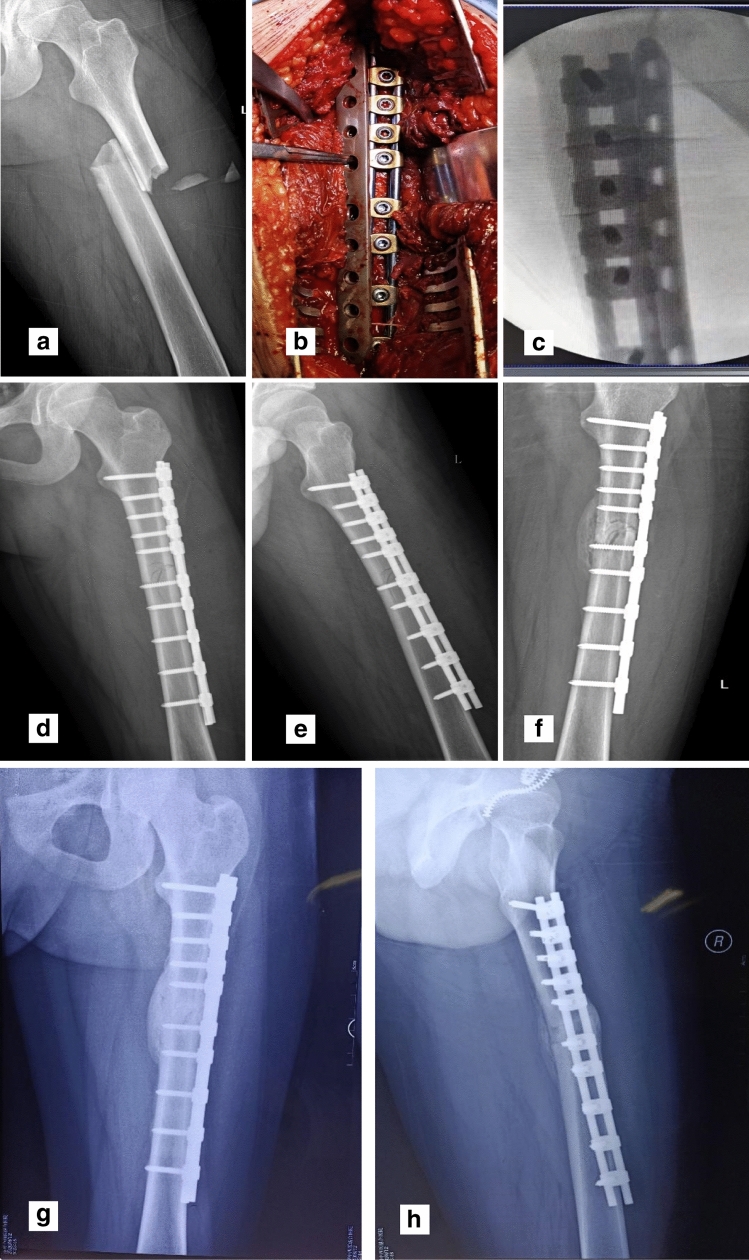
Typical case of a femoral fracture. a Preoperative X-ray analysis of B2 fracture of femoral shaft. b During the operation, the OBS sliding module is placed, and screws are inserted according to the distribution of nail holes of LCP. c Intraoperative C-arm fluoroscopy shows that the distribution of OBS screws is consistent with that of screw holes. d, e AP and LAT X-ray immediately after operation. f One month after the surgery, AP X-ray showed obvious callus growth of the contralateral side of OBS. g, h AP and LAT X-rays showed fracture healing at 3.5 months after surgery. *OBS* Ortho-Bridge system, *LCP* locking compression plate
